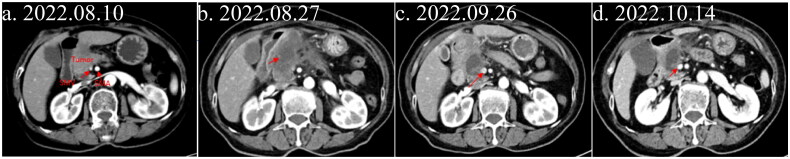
Abdominal imaging changes in pancreatic cancer during treatment with lenvatinib and icaritin. The red arrow indicates the tumor site. (a) Enhanced abdominal CT at admission. (b) Enhanced abdominal CT after initial treatment. (c) Enhanced abdominal CT at 40-day follow-up. (d) Enhanced abdominal CT at 2-month follow-up.
